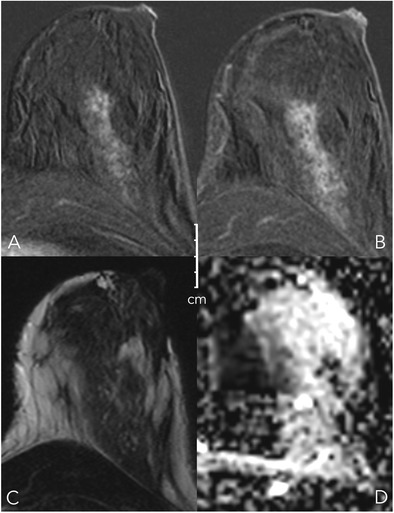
Early (A) and delayed (B) contrast-enhanced subtractions, T2w (C), and ADC map (D). Non-mass lesion in the left breast of a 25-year-old female with pain after stopping breast-feeding and unclear sonographic findings. The lesion shows no spiculations, a persistent enhancement curve type, and irregular margins, corresponding to a Kaiser score of 3 (Fig. 6). Note the internal small cystic correlate that suggests a benign lesion; ADC is unspecific (1.2*10^-3^ mm^2^/s). Together with the clinical symptoms, periductal mastitis must be suspected and it is safe to assign BI-RADS 2. Due to personal preferences, the patient requested a biopsy that revealed chronic periductal mastitis, B2
